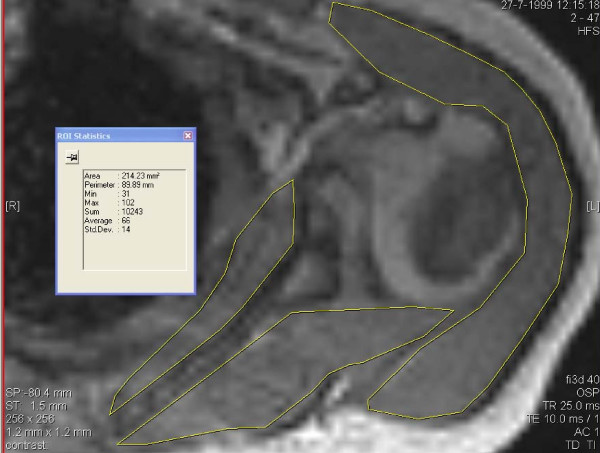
Transversal MR image of affected shoulder with area of 3 muscles outlined and showing Centricity 600 results of area measurement of subscapularis.
